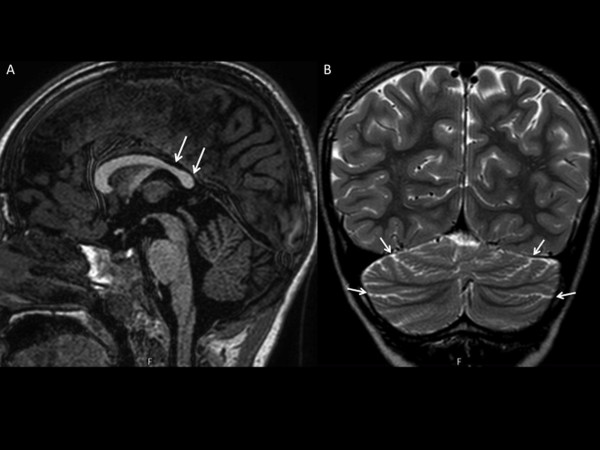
MR imaging. A) sagittal turbo-field-echo T1-weighted image shows hypoplastic callosal splenium (arrows). B) coronal fast spin-echo T2-weighted image shows slightly prominent cerebellar fissures (arrows), consistent with a mild form of cerebellar hypoplasia.
